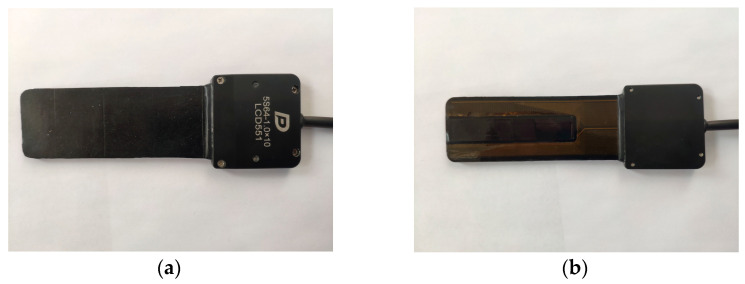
Doppler 5S64-1.0×10 multi-element flexible acoustic array. (a) Top view; (b) Bottom view.
